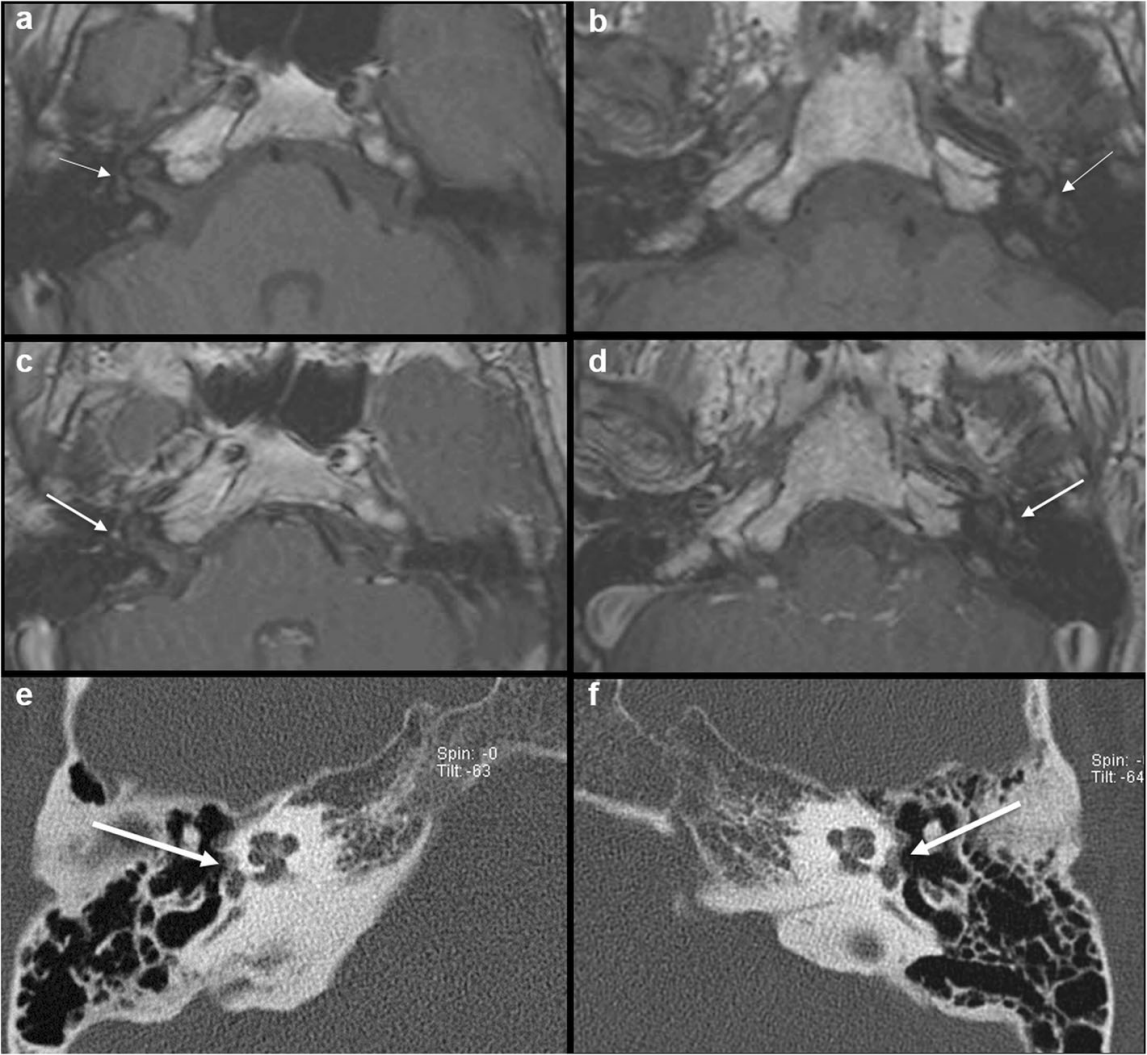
A 28-year-old male patient with left-sided SNHL (case 2). This was the case where otosclerosis was missed on first read MRI. Retrospective evaluation of axial T1W MR images (a, b) shows very subtle intermediate T1 signal in bilateral FA regions (arrows). Contrast-enhanced axial T1W images (c, d) show subtle focal post-contrast enhancement in bilateral FA regions (arrows). Axial HRCT images (e, f) show bilateral fenestral otosclerotic plaques (arrows). There was no pericochlear disease seen on HRCT
